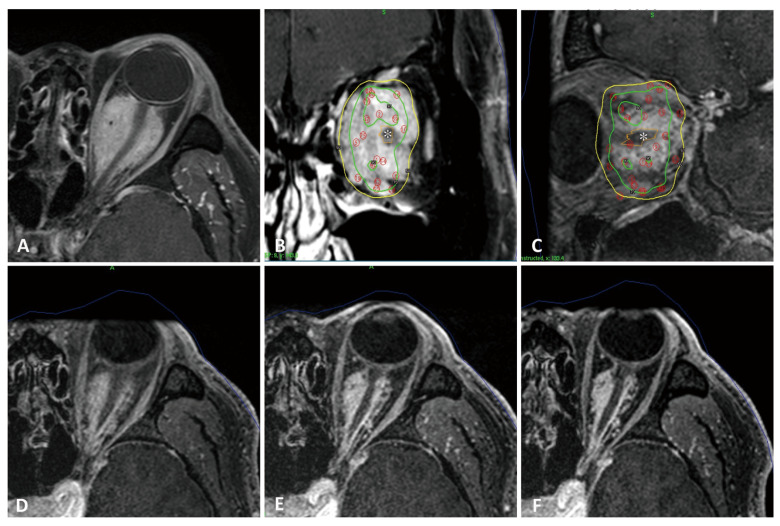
(A) Axial preradiosurgical MRI showing a meningioma encasing the left optic nerve. (B,C) Coronal (B) and sagittal (C) views of Gamma Knife dose planning; the optic nerve (white asterisk) is excluded from the 90% isodose line. (D–F) Follow-up MRIs at 6 months (D), 12 months (E), and 24 months (F) demonstrate marked tumor shrinkage.
